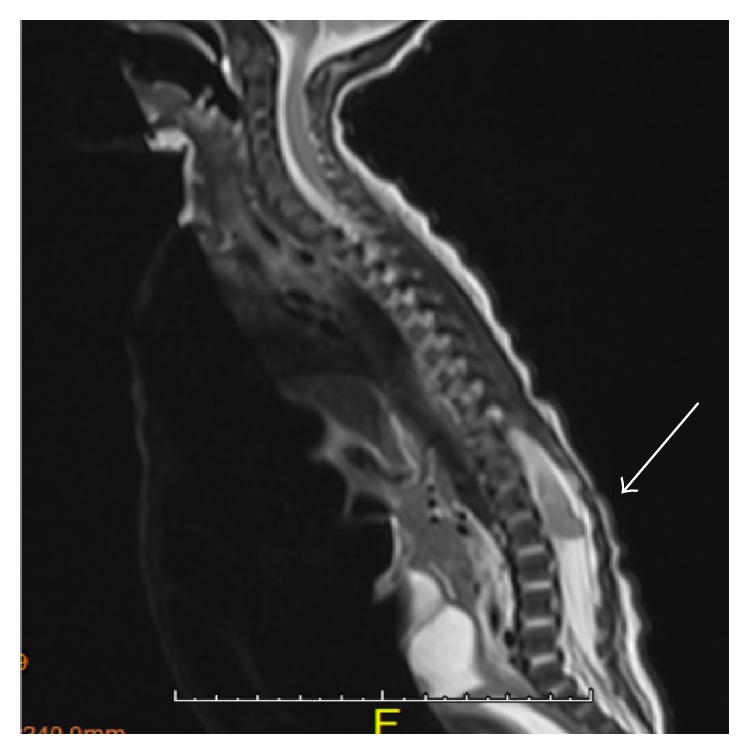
MRI spine, with and without contrast: expansile, somewhat lobulated mass in conus medullaris (see arrow).
